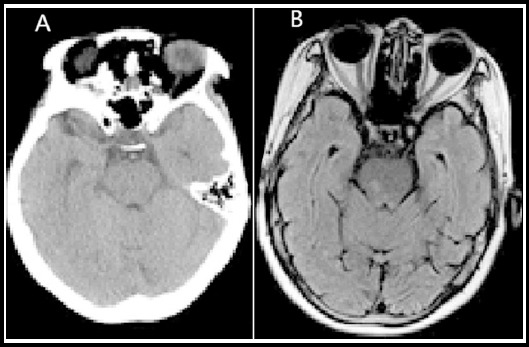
The lesions of patients with lacunar infarction in the examination with CT and MRI (A: Unclear display by CT; B: Clear display by MRI)
